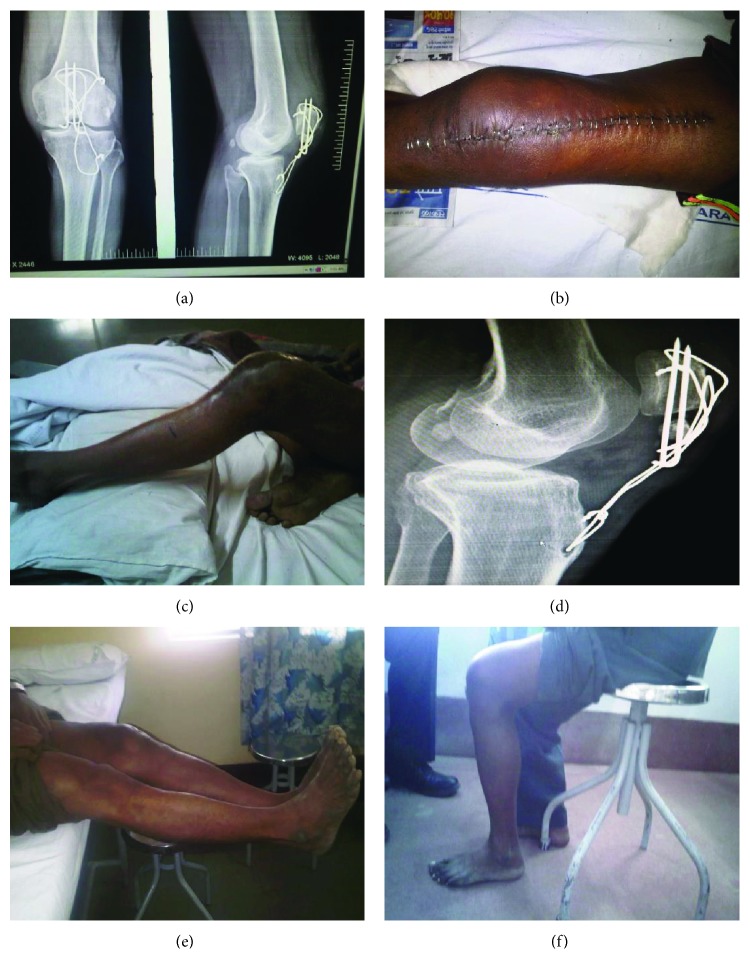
Postoperative X-ray, suture line, current X-ray, and present knee range of movement (a–f).
